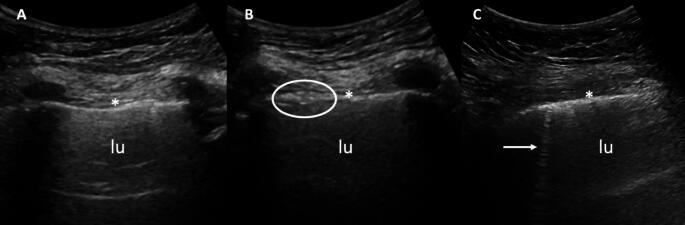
Ultrasound in lung involvement (interstitial lung disease). A shows a lung ultrasound using a convex probe, demonstrating a normal pleural line. B highlights pleural line irregularities, visible as nodular thickening (white circle), and C reveals a B-line (indicated by the white arrow) in patients with rheumatoid arthritis. *Asterisks* = pleural line; *LU* = lung parenchyma
